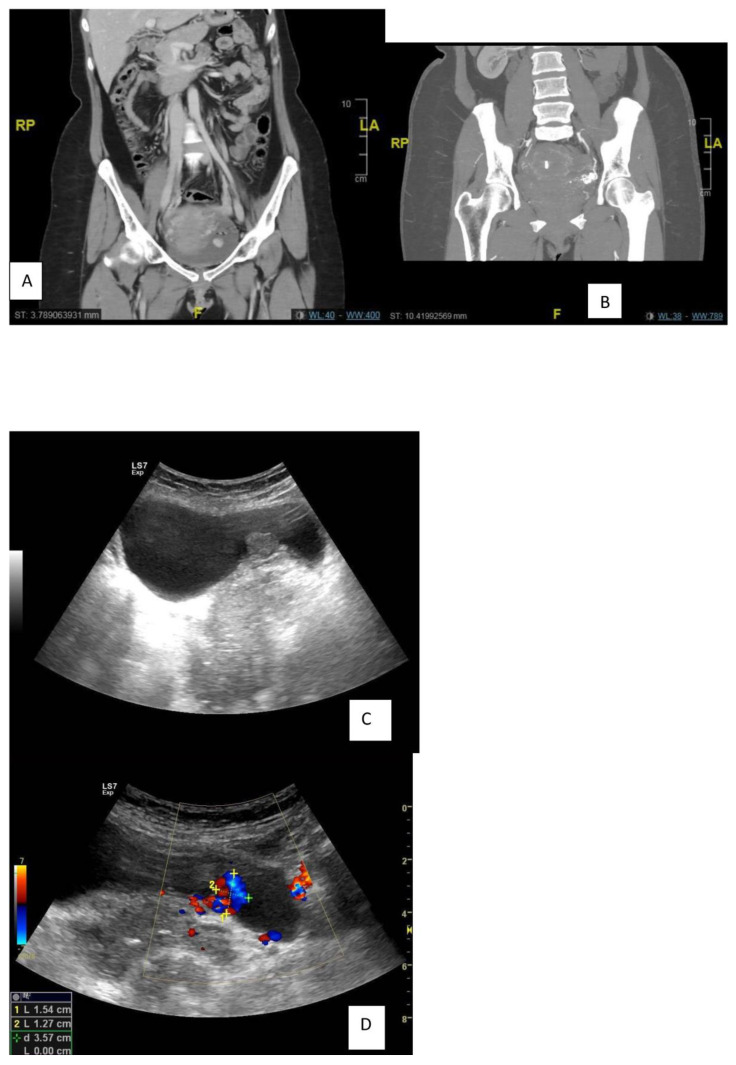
Recurrence after 5 years of follow-up. (A,B): CT showed a hypervascular lesion in the same site of the previously embolized AVM in the posterior urinary bladder wall (C,D): Follow up ultrasound Grey scale and color doppler.
